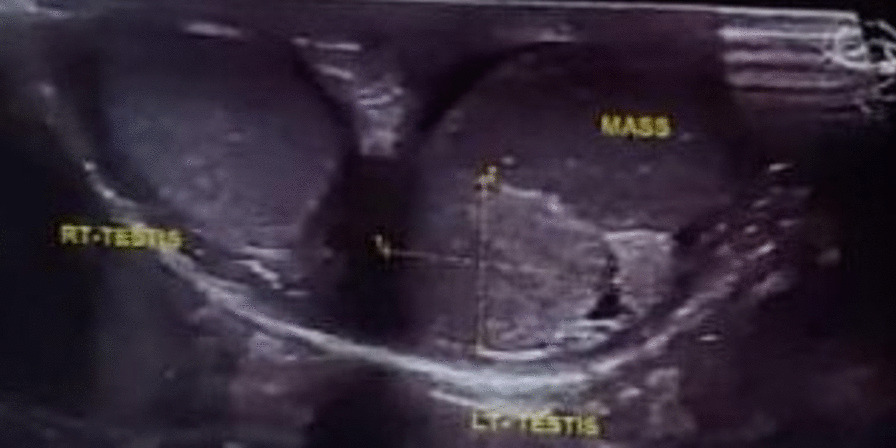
Ultrasound revealed hyper vascular mass lesion related to left epididymal head
